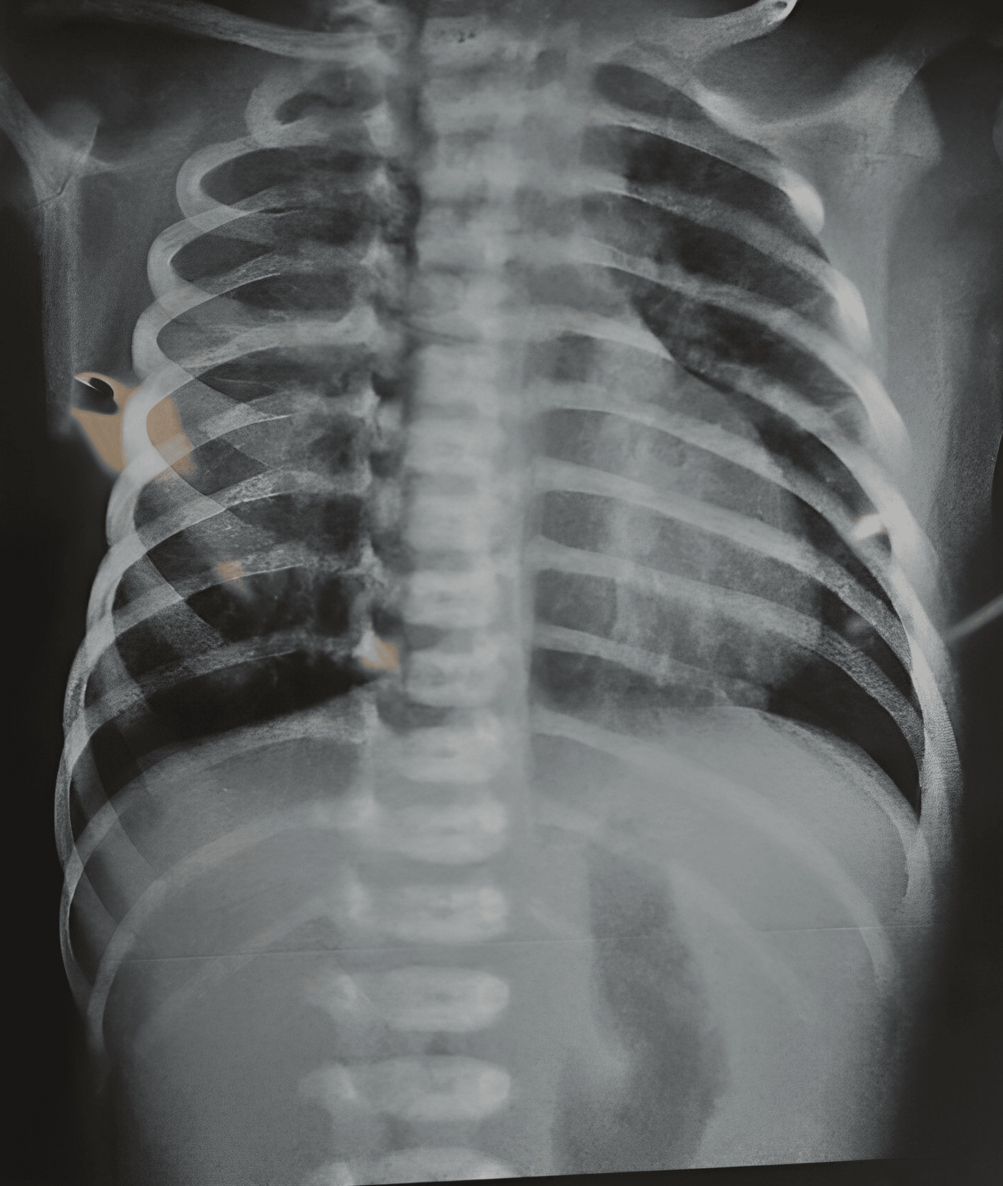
Chest X-ray on admission to the neonatal intensive care unit.
